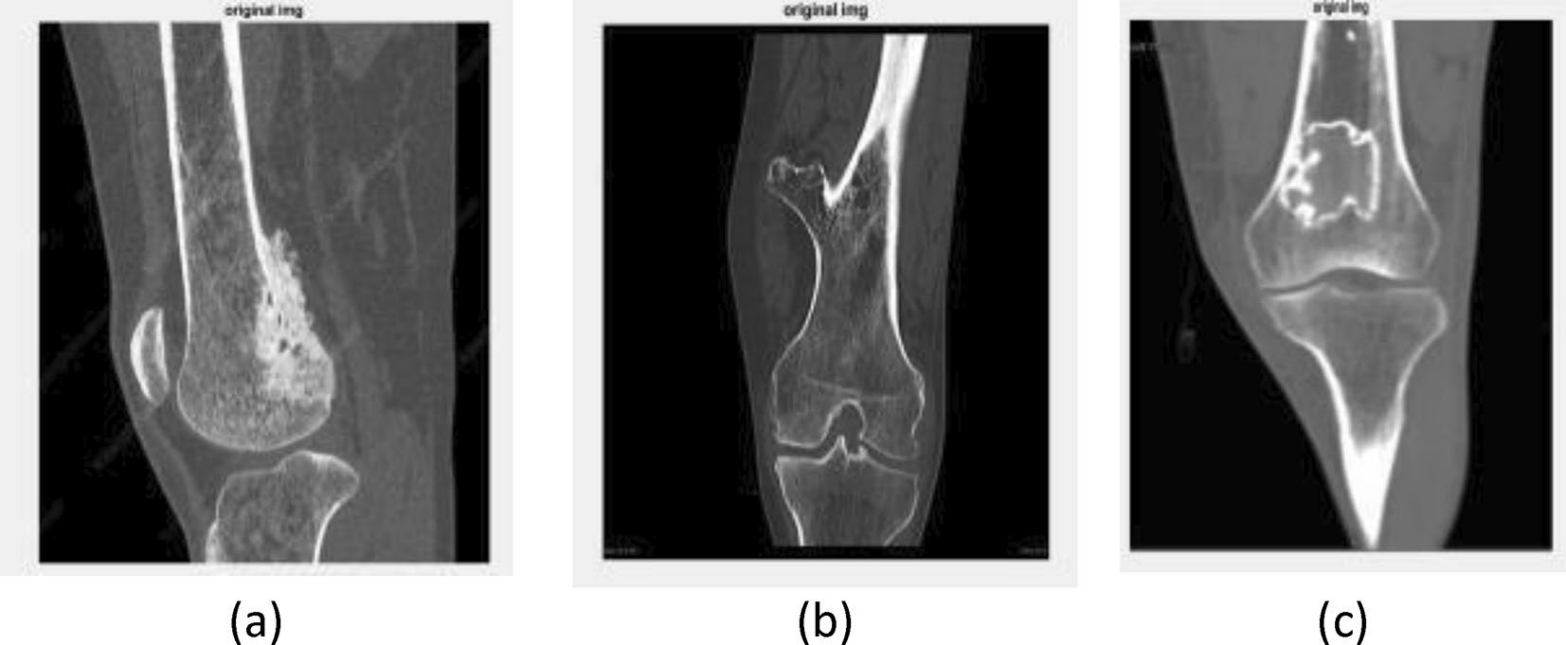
Original CT images: (a) lateral CT of parosteal osteosarcoma, (b) coronal CT of Osteochondroma, and (c) lateral CT of Enchondroma.
